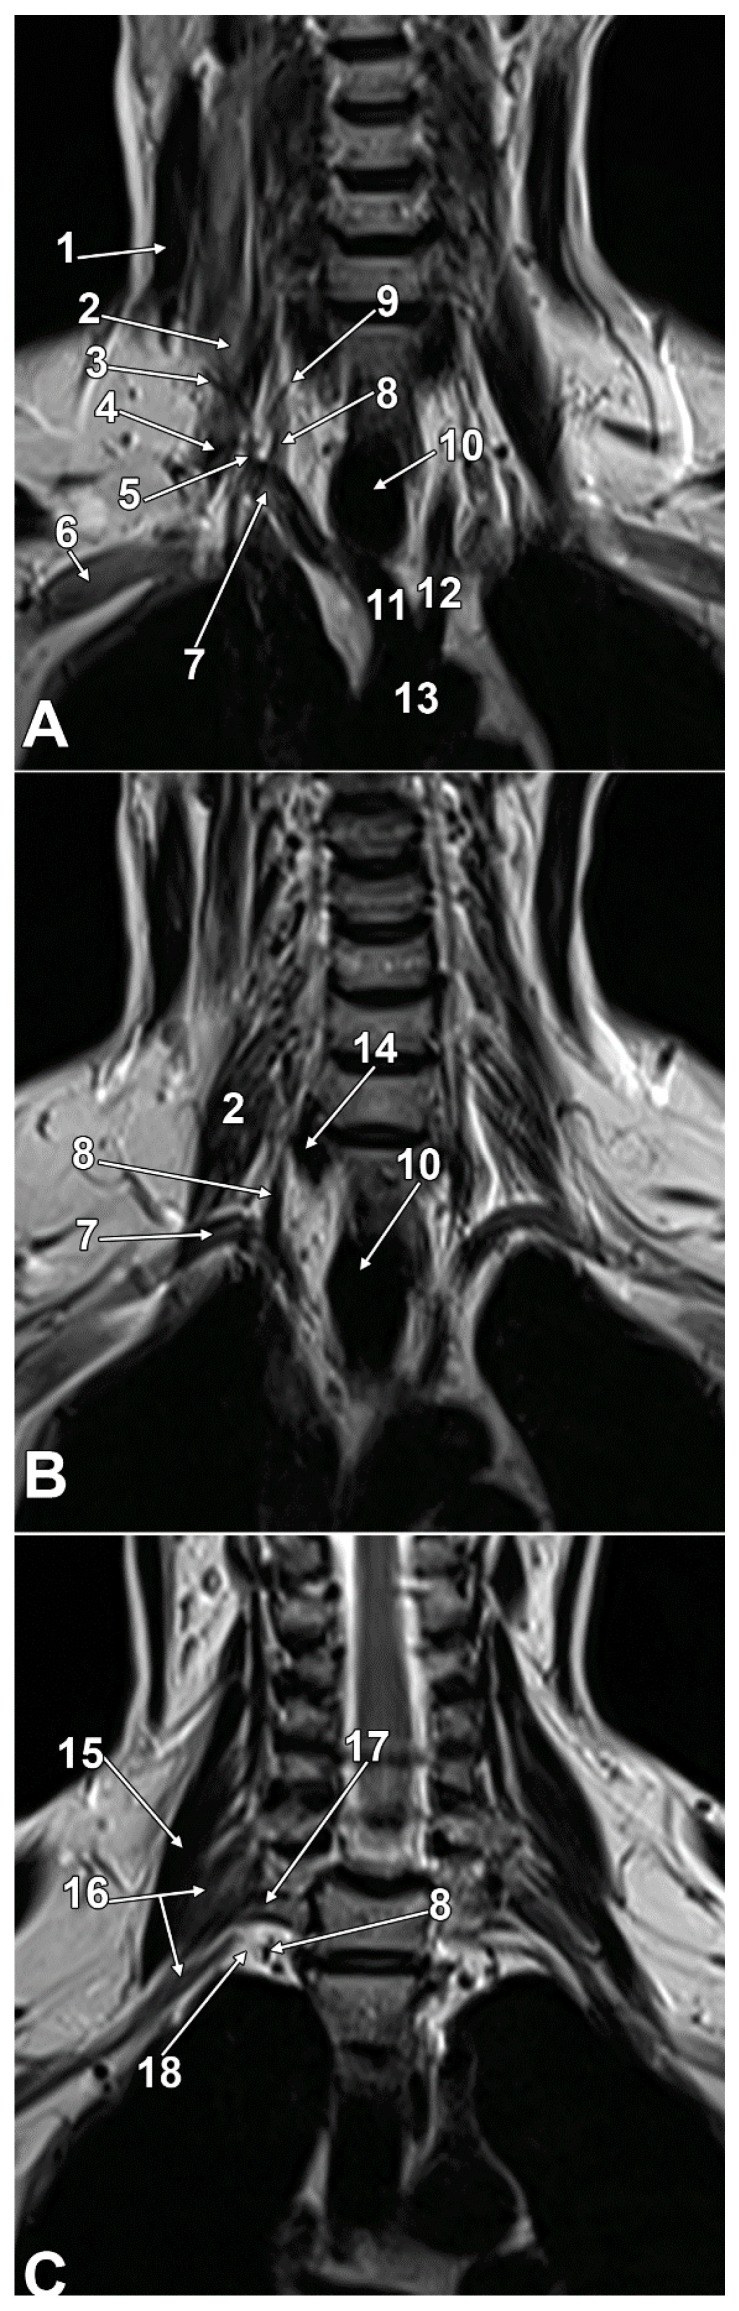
Successive anterior-to-posterior (A–C) original coronal MRI slices through the cervicothoracic region. Anterior view. Magnetic resonance imaging was performed using a 1.5 Tesla MRI scanner (Siemens Magnetom Avanto). Coronal plane T1-weighted turbo spin-echo imaging of the cervicothoracic junction was acquired with the following parameters: sequence: T1-weighted turbo spin-echo (TSE), repetition time (TR): 550 ms, echo time (TE): 12 ms, slice thickness: 3 mm, inter-slice gap: 0.3 mm, FOV: 300 mm, matrix: 384 × 384. Images were displayed using standard soft tissue window settings optimized for visualization of neural structures, muscles, and vascular anatomy. The stellate ganglion (18) was identified as an intermediate signal intensity structure at the thoracic inlet, located anterior to the neck of the first rib (17), lateral to the longus colli muscle (14), and posterior to the vertebral artery (8). The ganglion’s position relative to surrounding vascular structures (subclavian artery, vertebral artery) and bony landmarks (C7 transverse process, first rib) was clearly delineated. 1. sternocleidomastoid muscle; 2. anterior scalene muscle; 3. transverse cervical artery; 4. suprascapular artery; 5. thyrocervical trunk; 6. axillary vein; 7. right subclavian artery; 8. vertebral artery; 9. inferior thyroid artery; 10. trachea; 11. brachiocephalic trunk; 12. left common carotid artery; 13. aortic arch; 14. longus colli muscle; 15. middle scalene muscle; 16. posterior scalene muscle; 17. neck of the first rib; 18. stellate ganglion.
